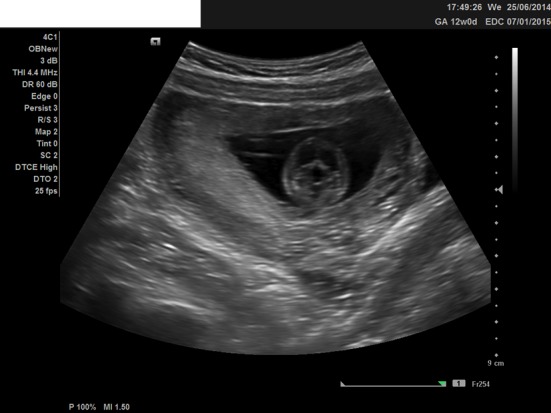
Ultrasound image at 12 weeks, showing the encephalocele, transverse view.
